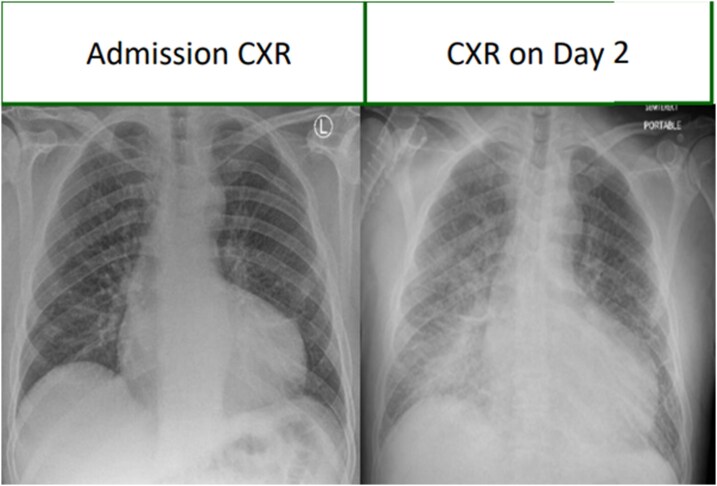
Chest radiograph demonstrating the development of consolidation.
